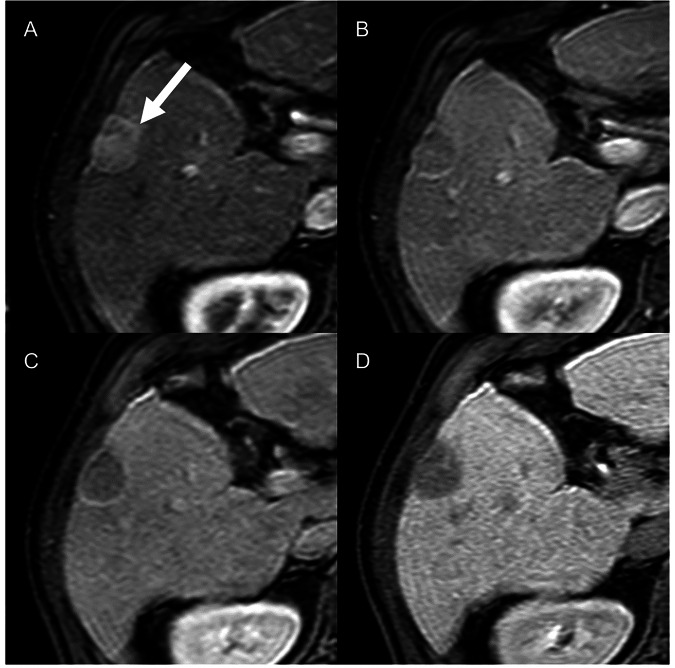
65-year-old male with hepatitis C-related cirrhosis. Gadoxetate disodium MRI demonstrates a 27 mm lesion in segment 5, with nonrim arterial phase hyperenhancement (A, arrow), nonperipheral washout and enhancing capsule on portal venous phase (B), hypointensity on transitional (C) and on hepatobiliary (D) phases as ancillary features favor malignancy. The lesion is categorized as LR-5 (definitively HCC)
